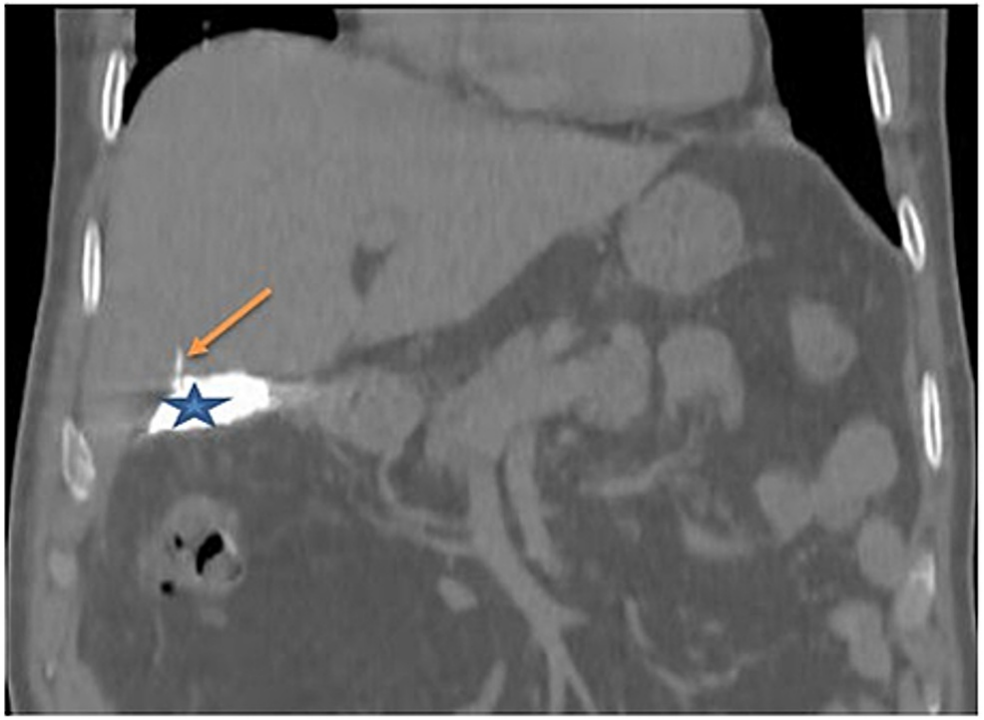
CT scan after drain opacification - view 2 The image shows opacification of the vesicular bed (blue star) and a small intrahepatic bile duct at segment V, which is connected to the injured duct of Luschka (orange arrow) CT: computed tomography
